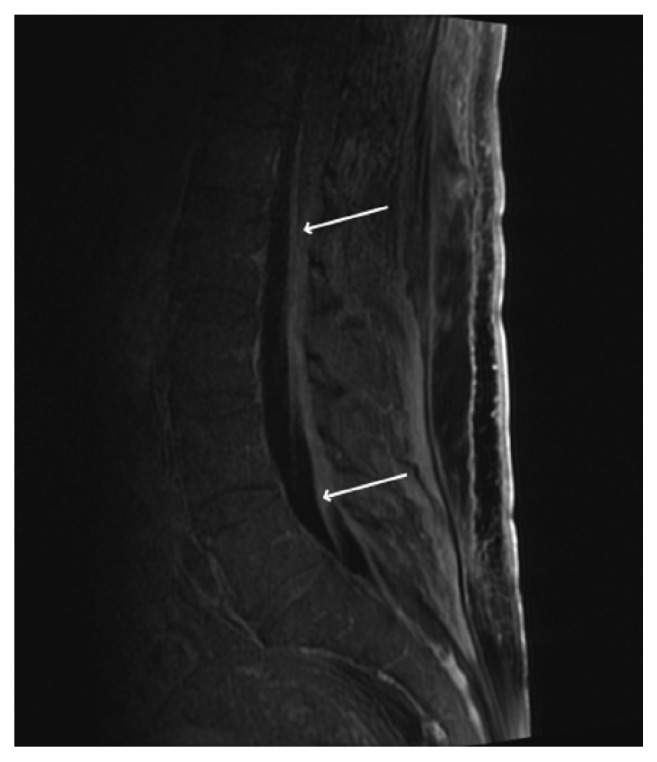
Sagittal view of MR lumbar spine T1 FLAIR depicting significant leptomeningeal enhancement and thickening of nerve roots (arrows).
